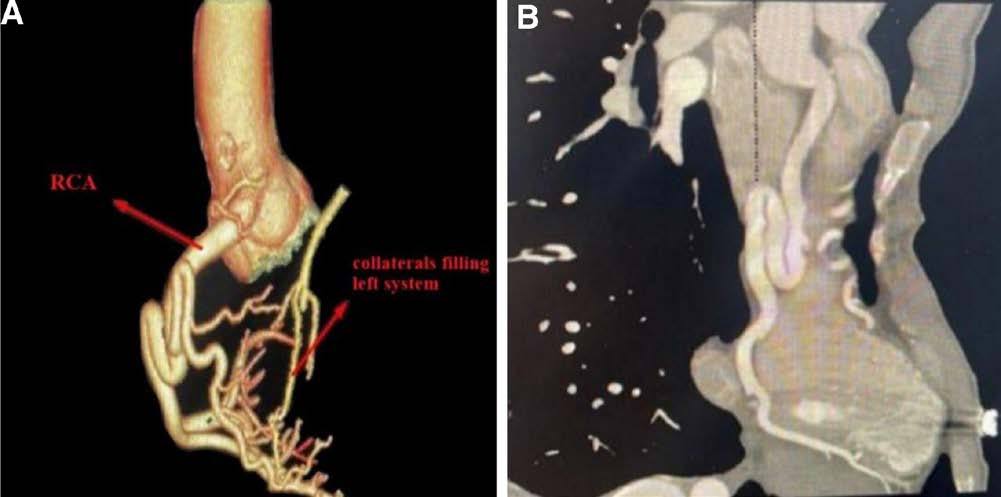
(A) Three-dimensional reconstruction of CT angiography images of the thoracic aorta. (B) Reconstruction for CT angiography of the aorta showing left main stem artery arising from pulmonary artery. CT = computed tomography.
